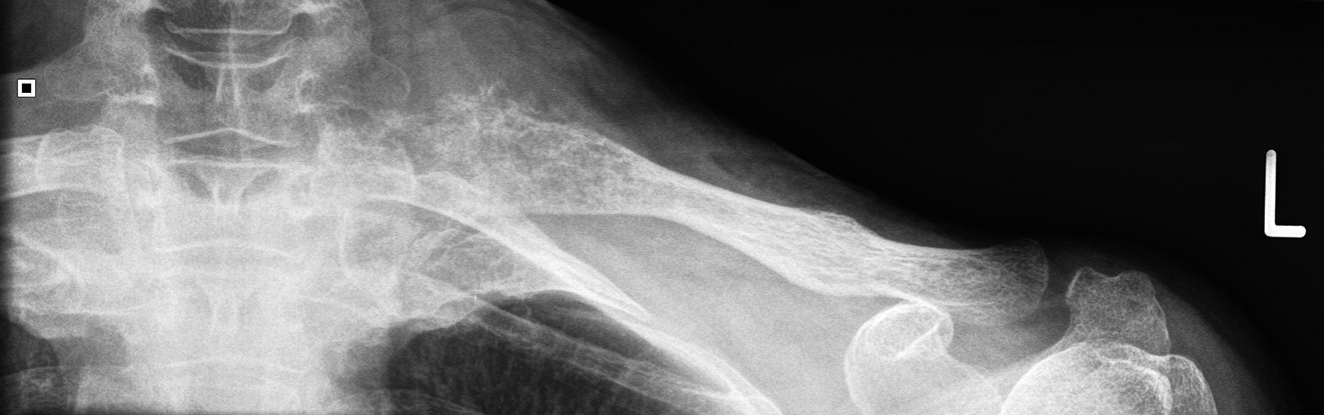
Radiograph showing destructive bony lesion of the medial left clavicle.
